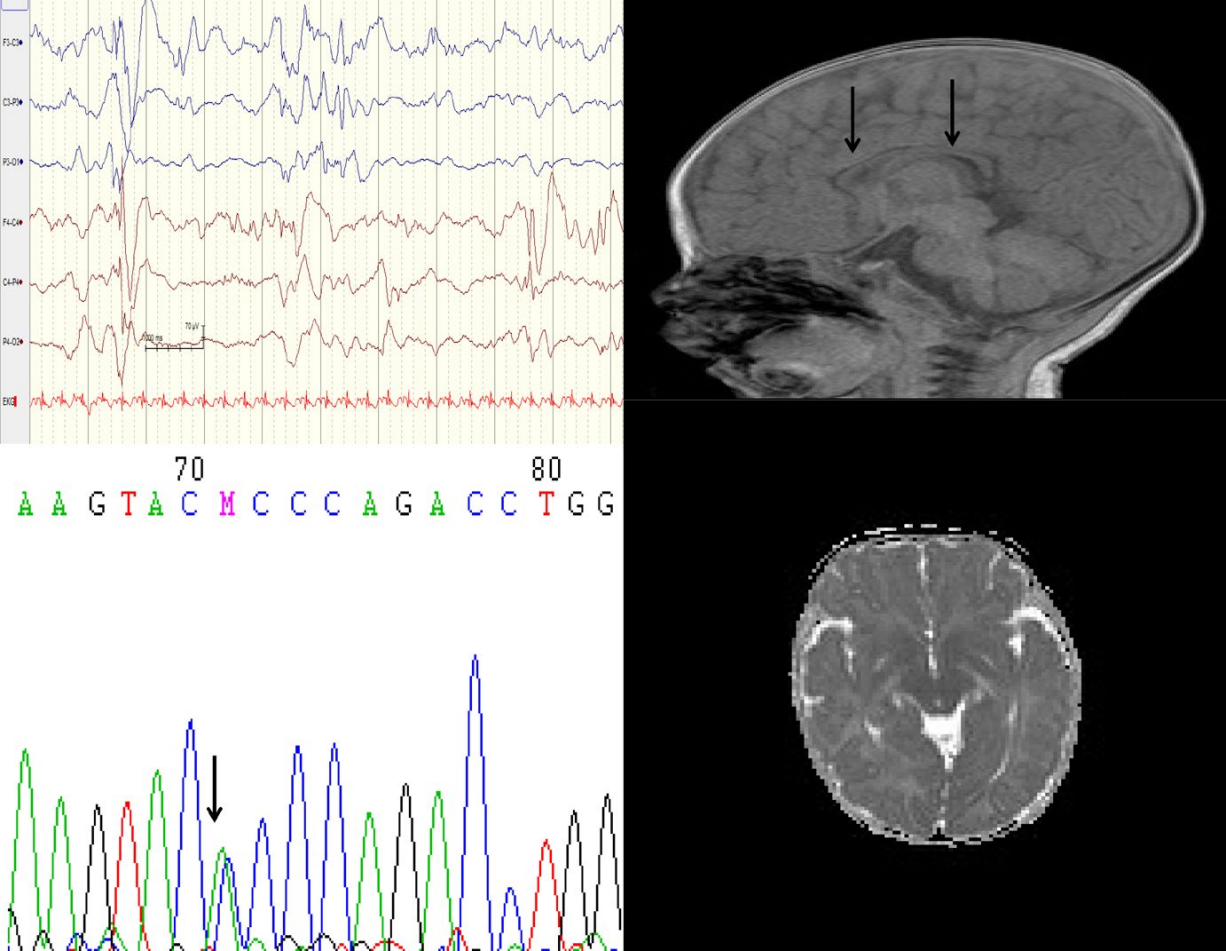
Patient 6 has a de novo c.853C>A p.(Pro285Thr) mutation (left lower) and an EEG that shows neonatal epileptic encephalopathy with burst‐suppression (left upper). Her EEG improved and her seizures attenuated after 2 months. The MRI shows a thin corpus callosum (right upper, arrows, and right lower)
